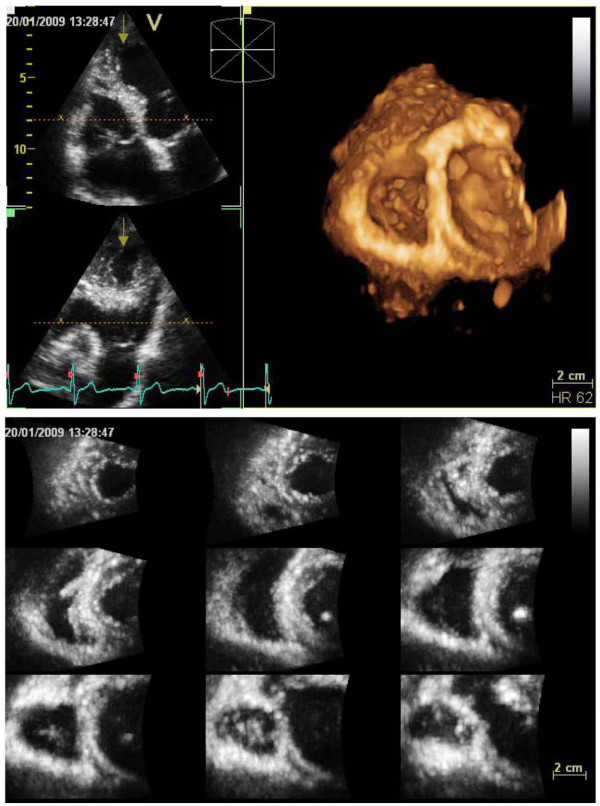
Real-time 3-D echocardiography for quantitation of the right ventricle. 2-D RV multiplane display and 3-D RV transversal plane during acquisition (upper panel) as well as 9-slice display after acquisition were used for quality check (lower panel).
